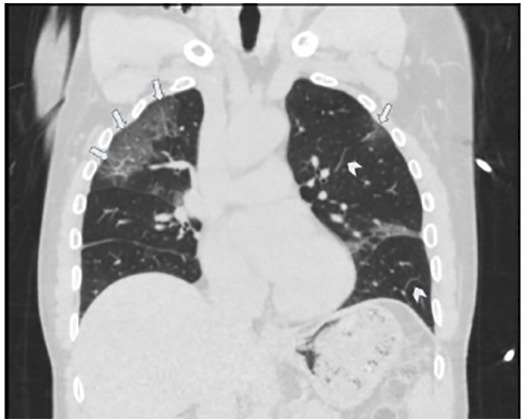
Chest computed tomography (coronal image) demonstrating multifocal peripheral bilateral ground glass opacities (arrows) with interlobular septal thickening (arrowheads) and mild peribronchial thickening, suggestive of infectious/inflammatory airway disease which can be seen in the setting of COVID-19.
